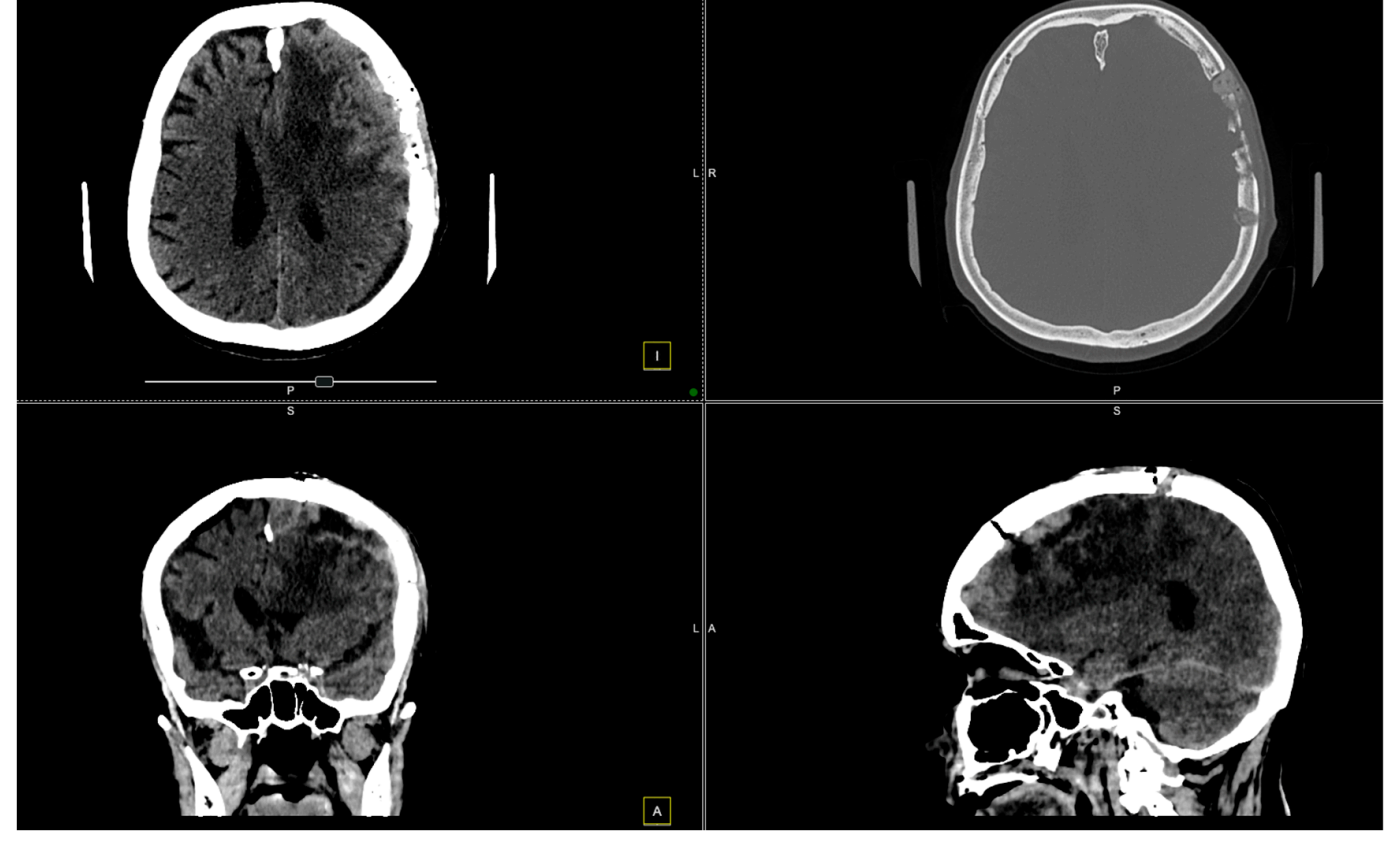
CT scan conducted 7 days post-surgery.
